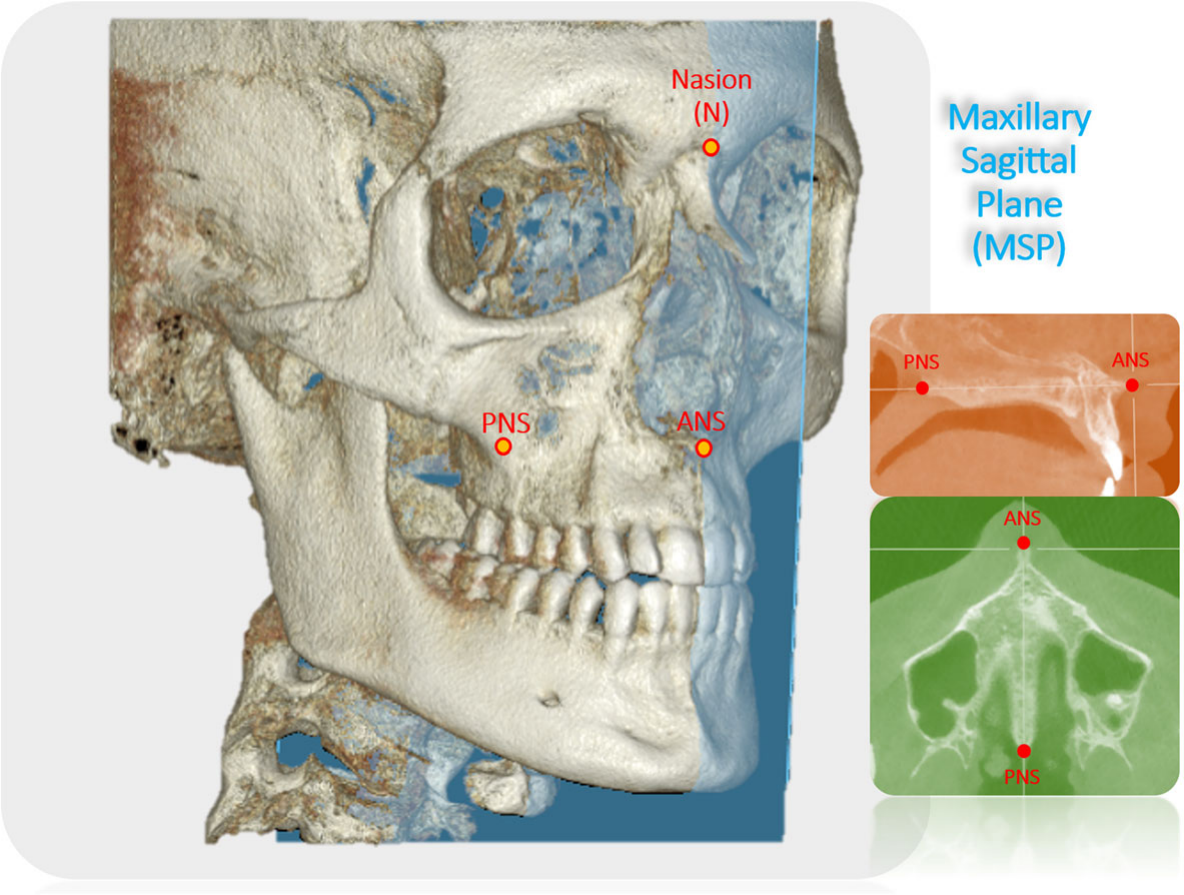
3D reconstruction with the maxillary sagittal plane (MSP) passing through anterior nasal spine (ANS), posterior nasal spine (PNS), and nasion (N) on the pre-expansion CBCT
